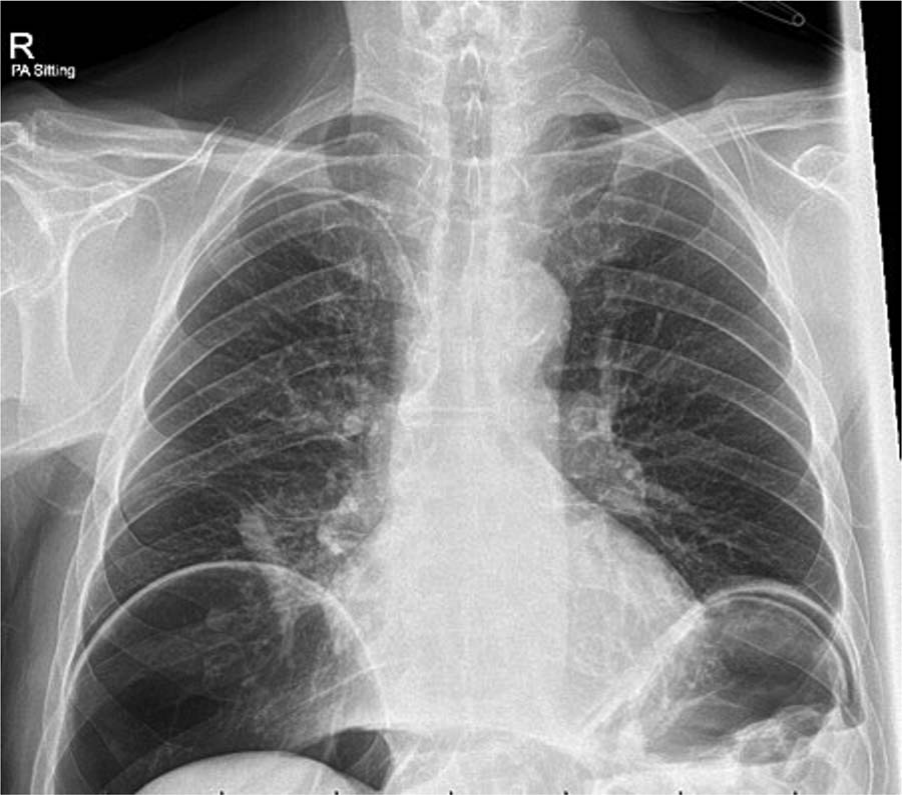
Day 10 chest x-ray demonstrating massive pneumoperitoneum.
